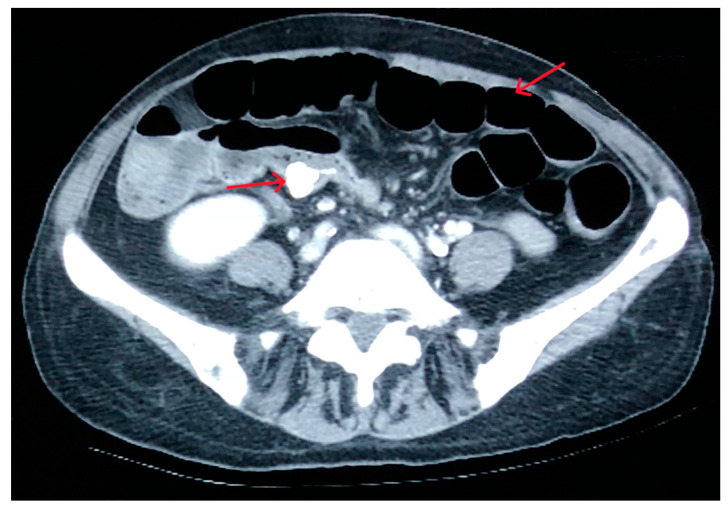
CT image with the foreign body (left arrow) and hydroaeric images (right arrow).
